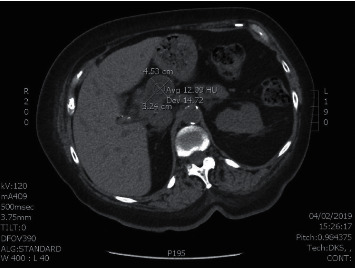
CT scan image of the abdomen without contrast showing the pancreatic mass before treatment.
